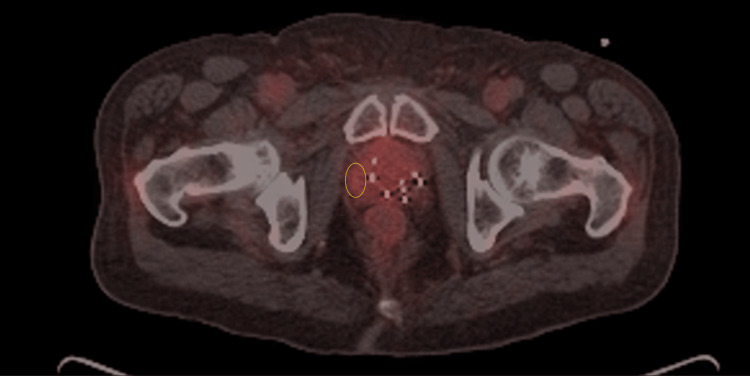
Pylarify PSMA PET showing recurrent disease in right lateral prostate The radiologist-defined PET-avid lesion is circled in yellow PSMA PET: prostate-specific membrane antigen positron emission tomography
